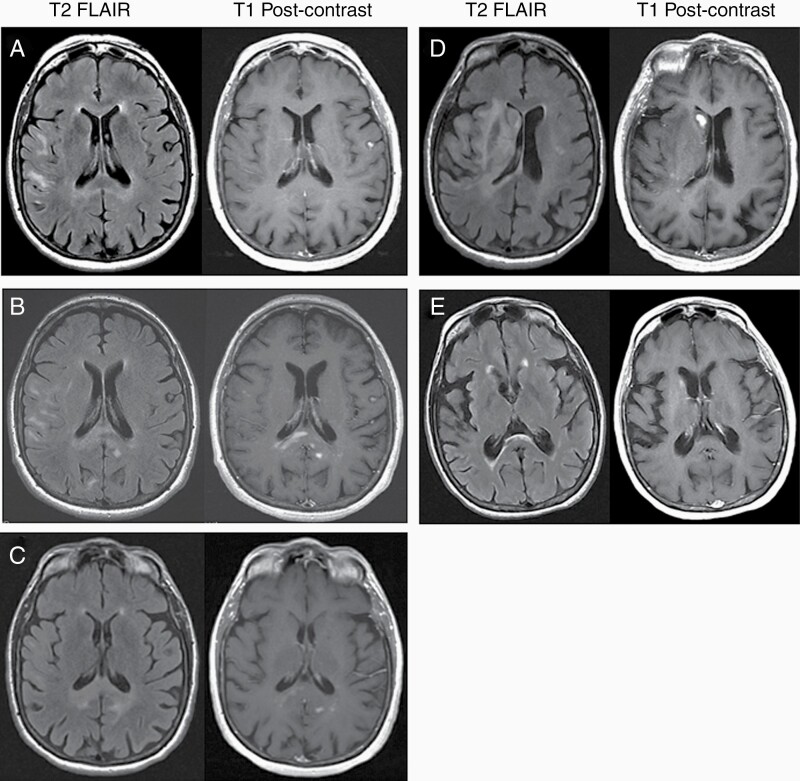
Changes in MRI brain over time. T2 FLAIR precontrast and T1 postcontrast images between initial presentation of symptoms and post-treatment with high-dose methotrexate and cytarabine consolidation. (A) Initial presentation, no contrast-enhancing lesions present. (B) One month after initial presentation, new contrast-enhancing lesions in the corpus callosum. (C) Post high-dose steroid administration, mild reduction in size of enhancing lesions. (D) Just prior to treatment with high-dose methotrexate. (E) Post-treatment with 8 high-dose methotrexate infusions and consolidation with cytarabine.
